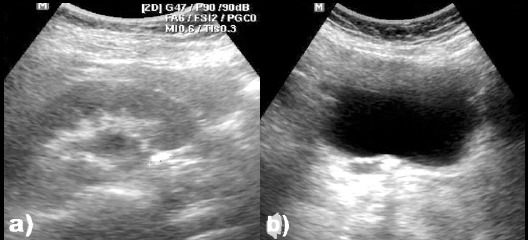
Ultrasound images for a) 10 mm stone in the right UPJ causing mild hydronephrosis, and b) Stone in the right UVJ causing hydroureteronephrosis.
